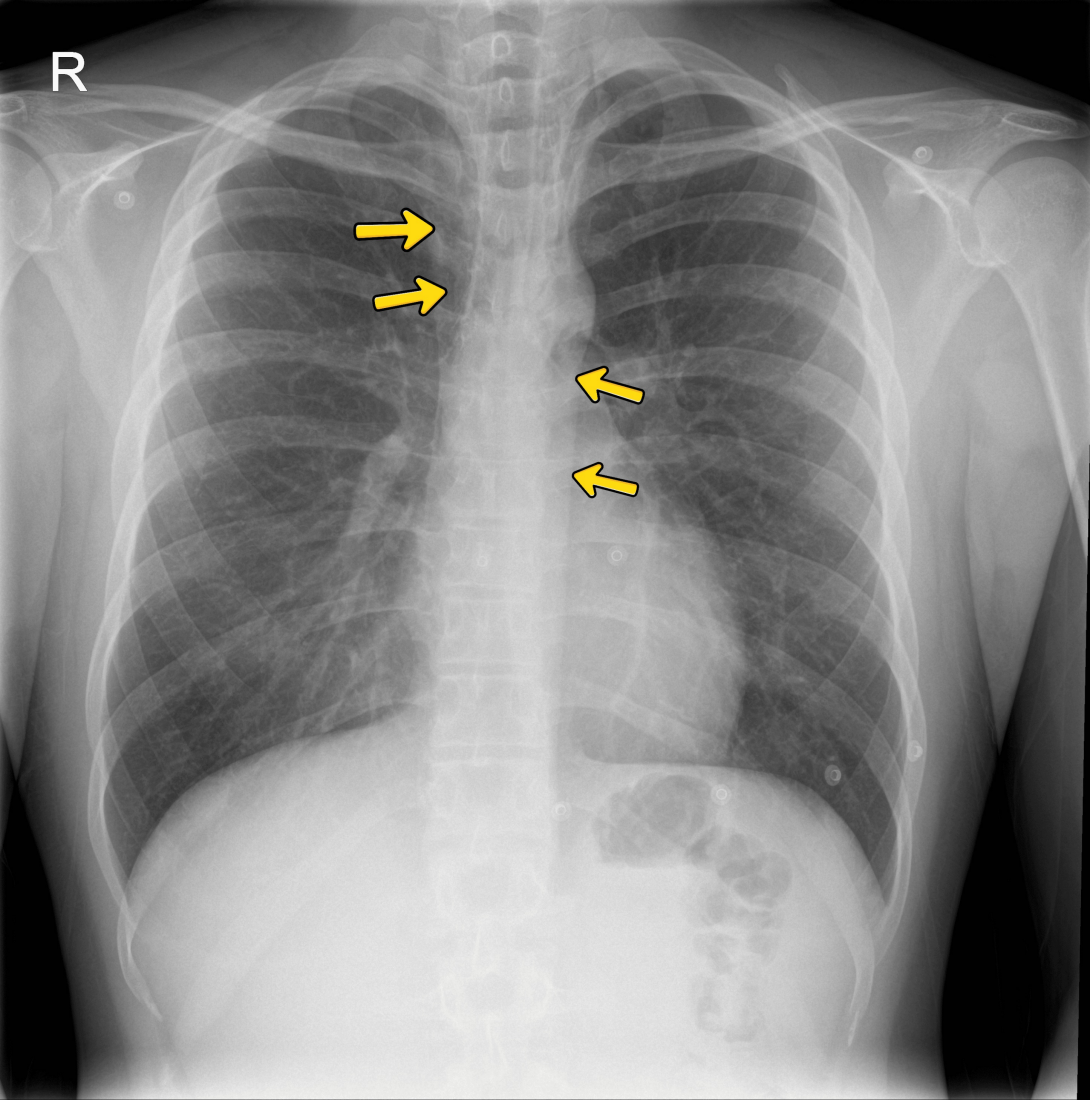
Chest radiograph showing pneumomediastinum
